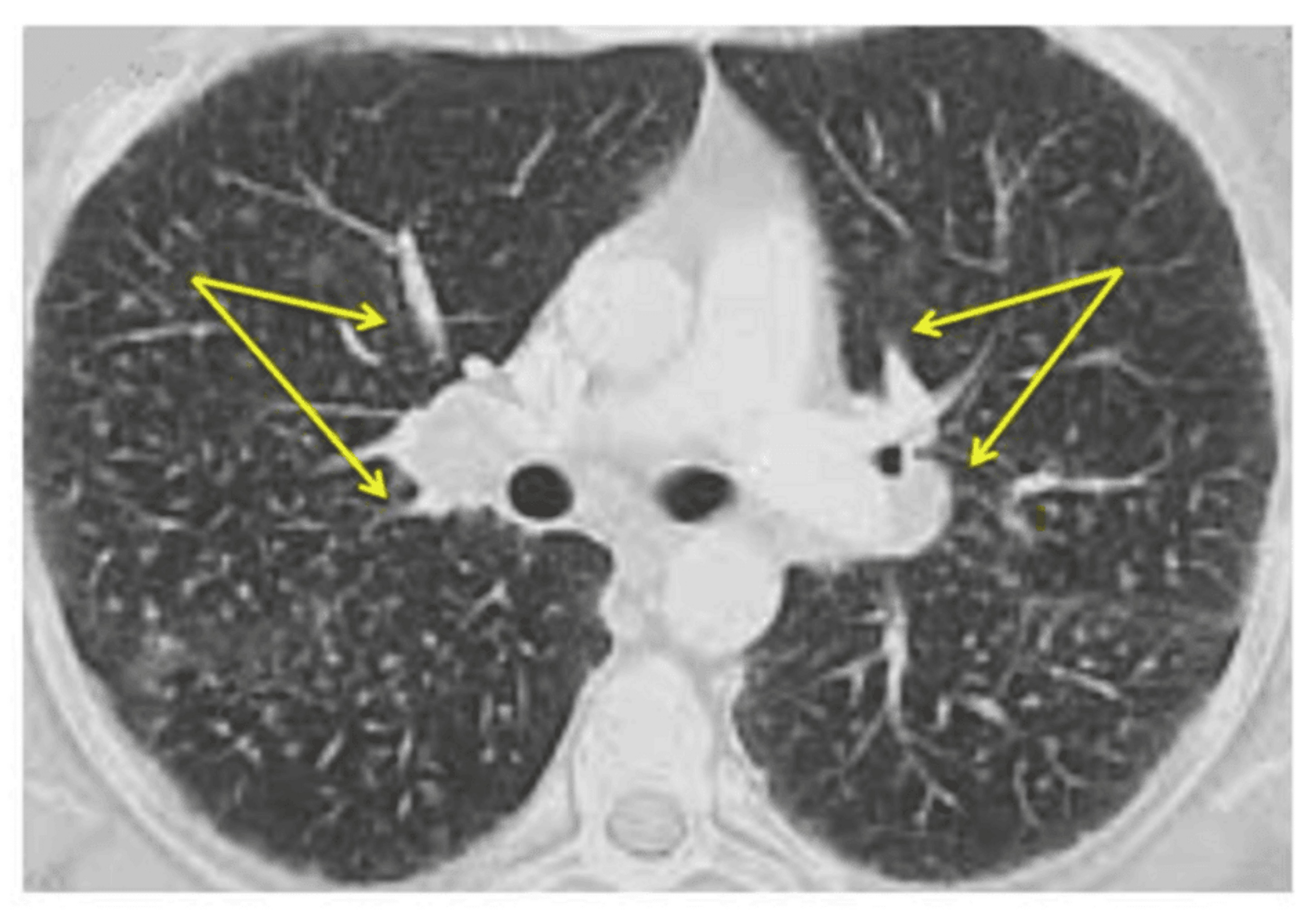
Thoracic CT scan findings The image shows bilateral, patchy interstitial and alveolar infiltrates with predominantly peripheral distribution (yellow arrows), suggestive of eosinophilic lung involvement CT: computed tomography
